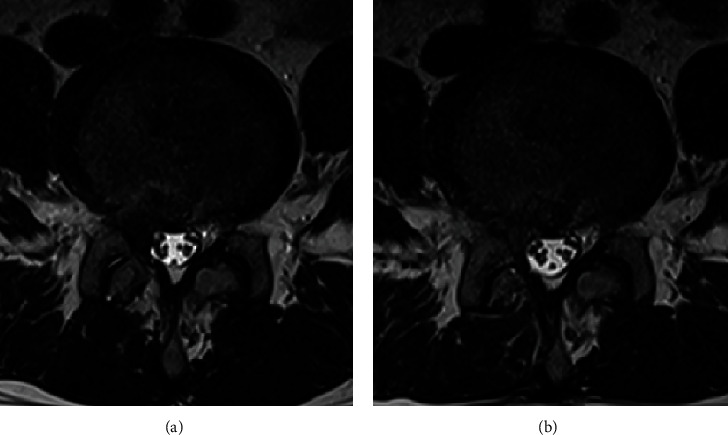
(a) T2-weighted MRI scan of a patient with disc herniation that recurred in the same area after L4–5 disc removal 20 years ago. (b) Postoperative T2-weighted MRI scan.
